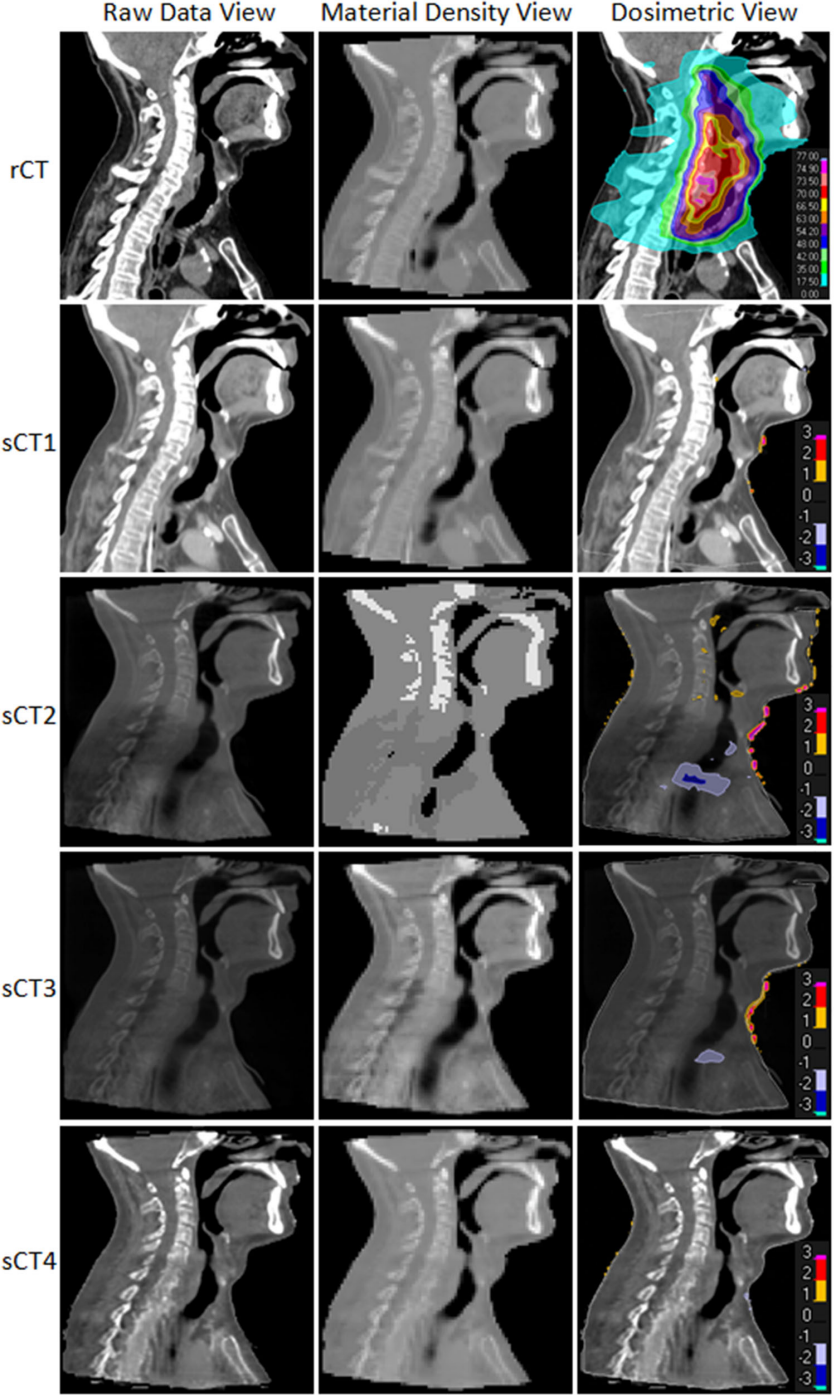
Three views of the rescan CT (rCT) and each synthetic CT (sCT) generated for a representative patient. The raw data view is typically used for contouring and general viewing of the image data. The material density view shows the mass densities on the dose grid. The dosimetric view shows the plan dose distribution for the rCT and the percentage dose difference (range −3%–3%) for the sCTs generated (normalized by the rCT dose). Positive values indicate a region is underdosed relative to the rCT and vice versa. sCT1—pCT deformable image registration (DIR) to dCBCT. sCT2—bulk density assigned dCBCT. sCT3—patient‐specific Hounsfield units (HU) calibration curve applied to dCBCT and low‐frequency artifacts removed. sCT4—trained machine learning model
